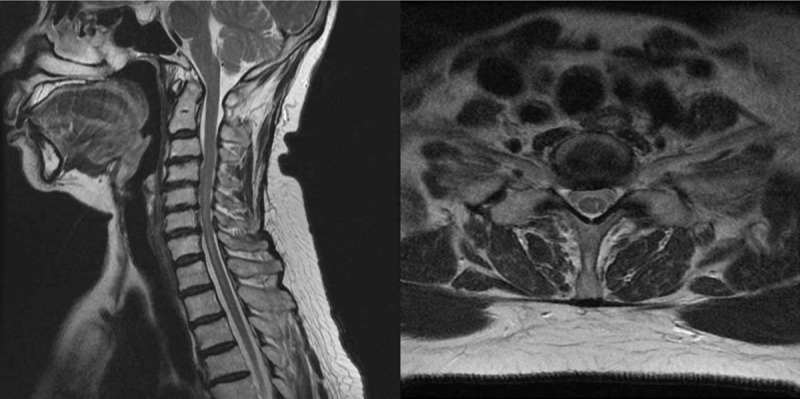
(A) Sagittal T2-weighted image of the cervical spine, the interval much decreased in the axial extent of the intramedullary high signal intensity at the left side spinal cord from C4 to T3, with decreased edema. (B) axial T2-weighted image of the cervical spine, showing decreased in the axial extent of the intramedullary high signal intensity.
